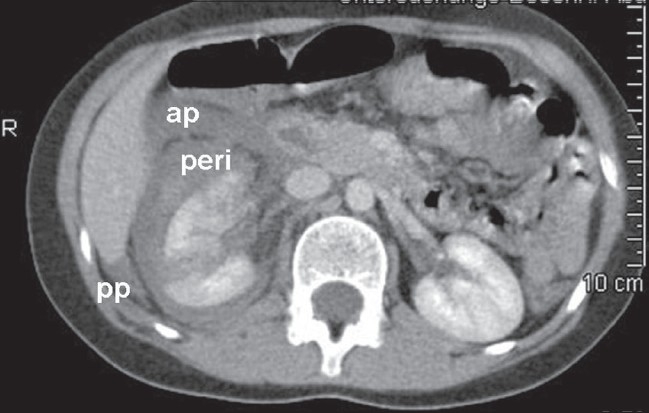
Peri- and pararenal hematoma after traumatic renal rupture (CT Scan)
